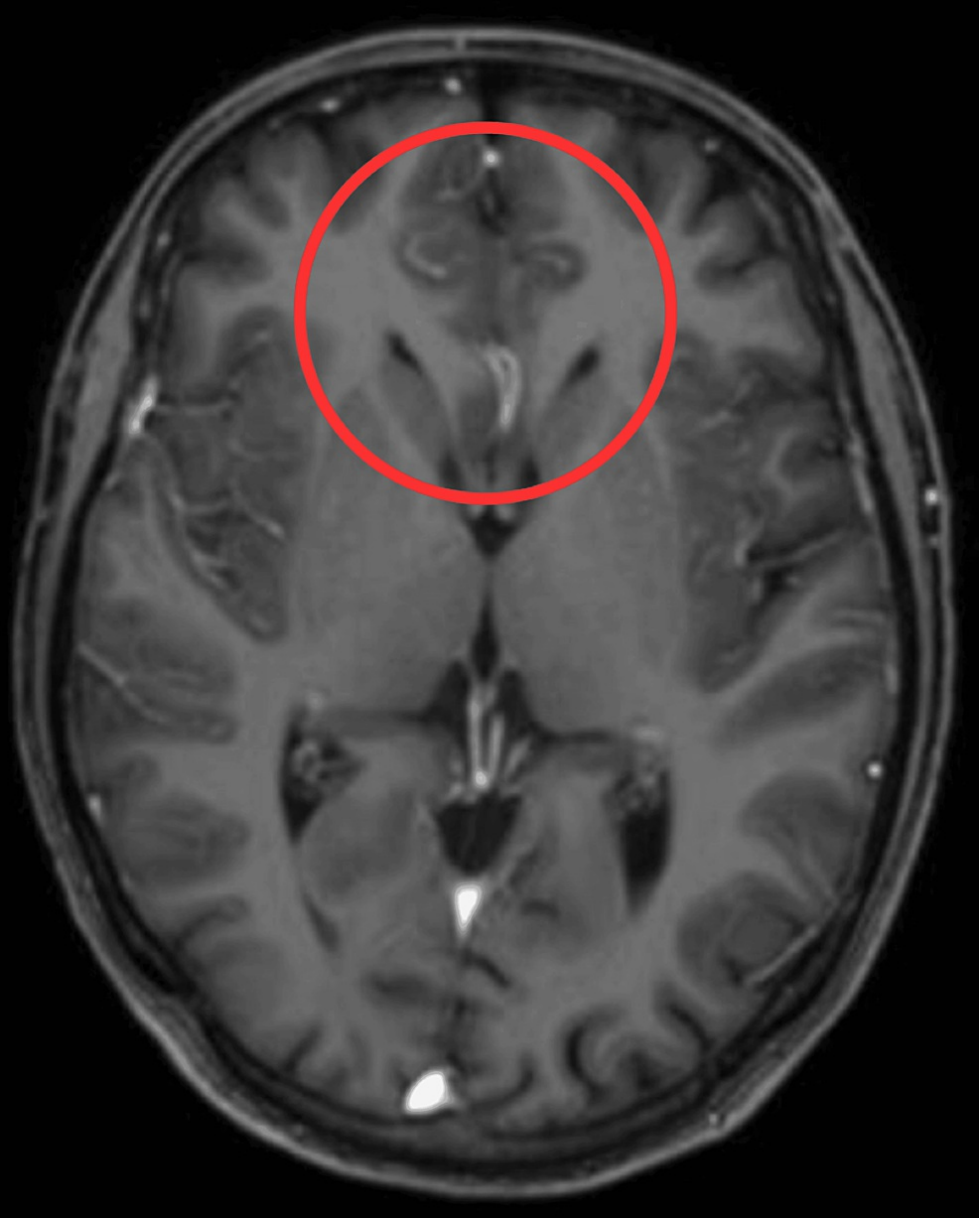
Magnetic resonance imaging of the brain. The red circle shows the non-enhancing symmetrical signal abnormalities with diffusion restriction in the bilateral parasagittal frontal region involving the cingulate gyrus.
